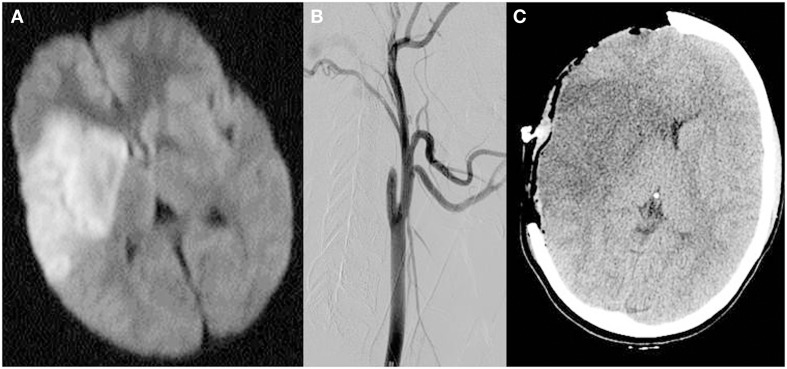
MRI diffusion-weighted images demonstrating restricted diffusion in the right middle cerebral artery territory including the basal ganglia (A), catheter angiogram demonstrating occlusion of the internal carotid artery distal to the bifurcation (B) and non-contrast head CT scan performed post-hemicraniectomy demonstrating large area of hypodensity in the right middle cerebral territory with mass effect (C).
